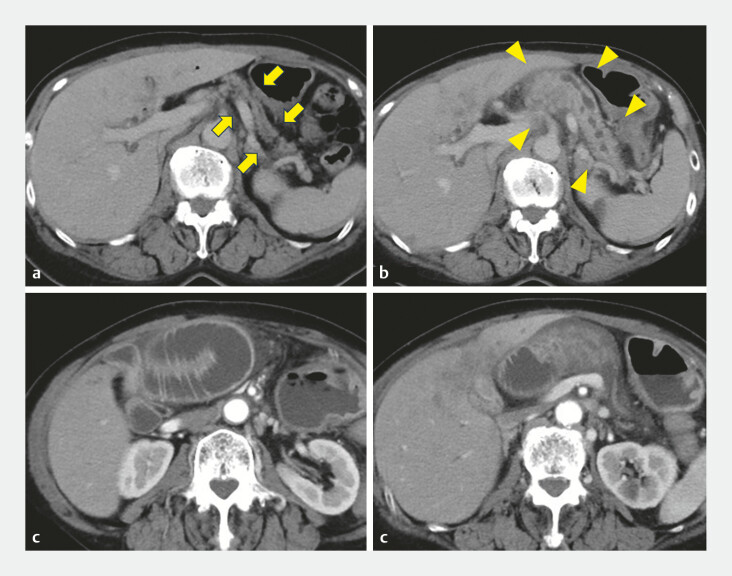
Computed tomography performed 3 months before admission and at the time of hospitalization:
